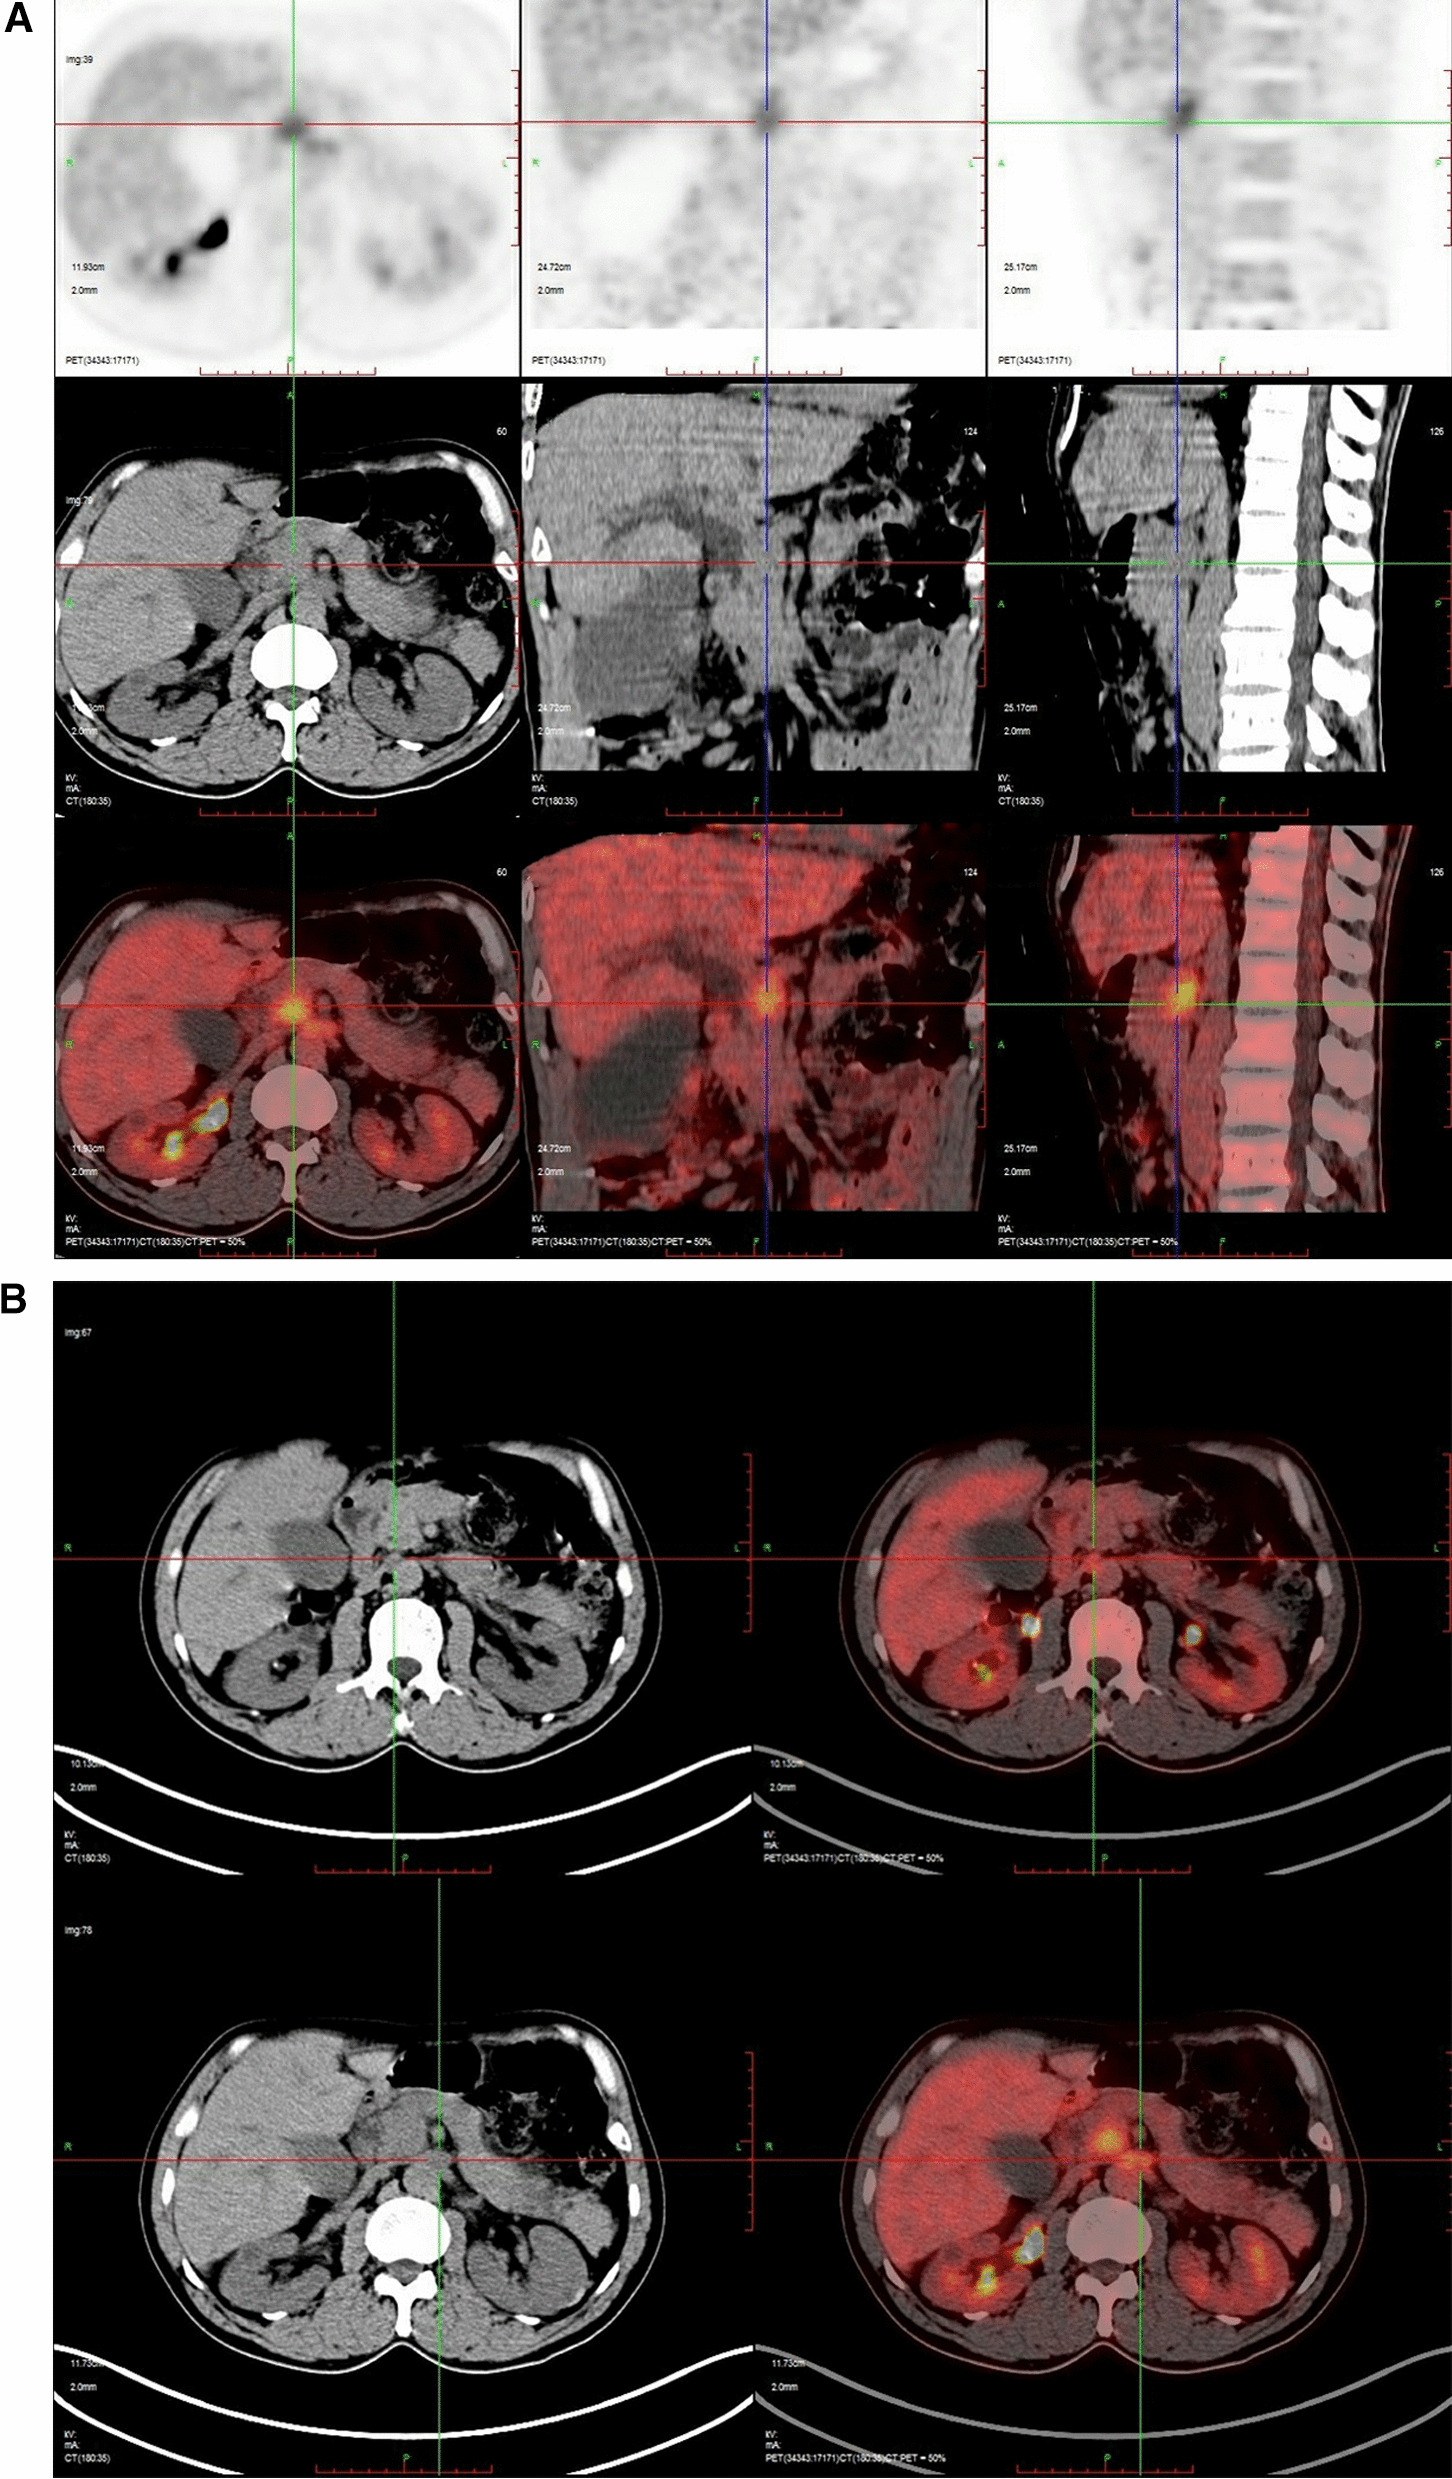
Positron emission computed tomography findings. a The round mass of the head of the pancreas exhibited posterior protrusion, with increased tracer distribution (SUVmax 6.91). The lesion involved the adjacent common bile duct and no abnormality was observed in the remaining pancreas. b Two abnormal perfusion foci in the retroperitoneum (behind the head of the pancreas) were closely attached to the abdominal aorta and left renal vein (SUVmax 4.45)
